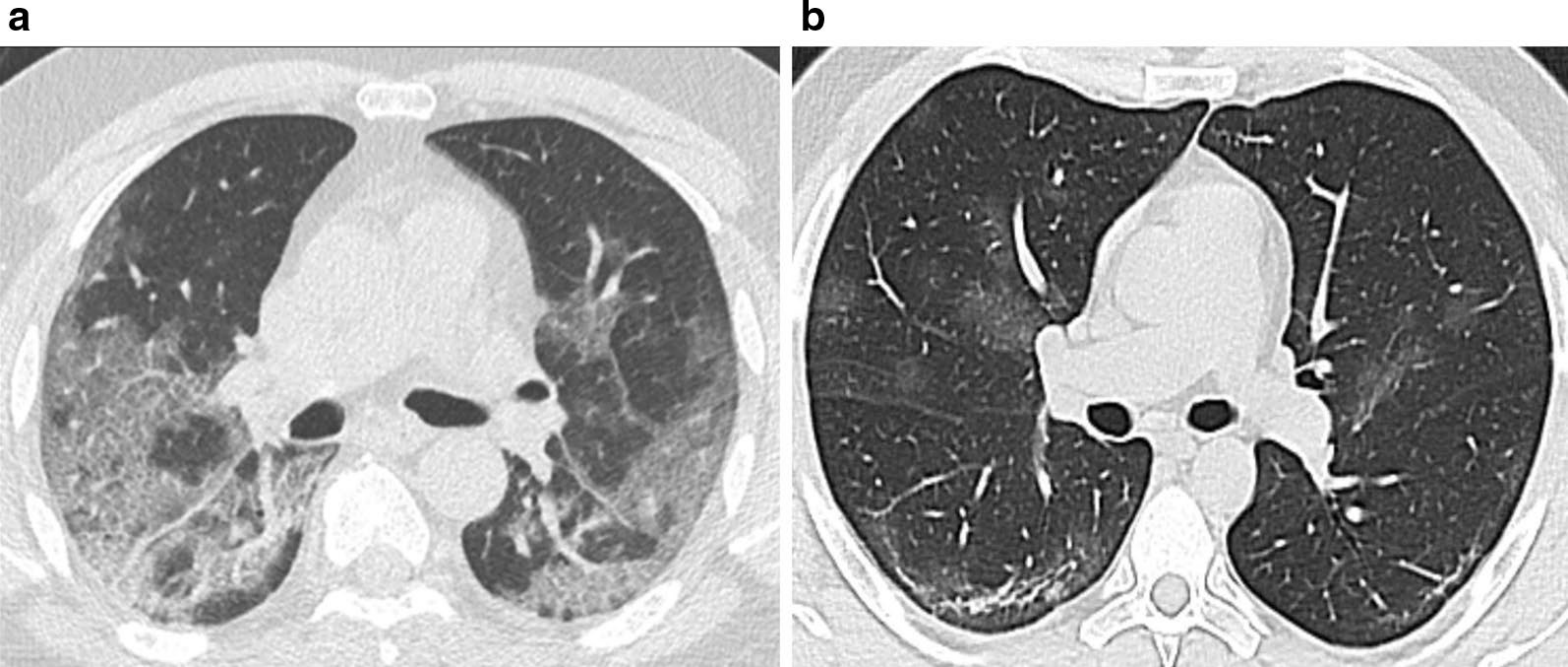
Typical CT imaging features for COVID-19. a A 72-year-old woman with positive RT-PCR test, axial CT image shows multifocal peripheral GGO with superimposed interlobular septal thickening and visible intralobular lines (“crazy-paving”). b A 46-year-old man with a positive RT-PCR, axial CT image shows bilateral multifocal rounded and peripheral GGO with bilateral posterior subpleural bands
